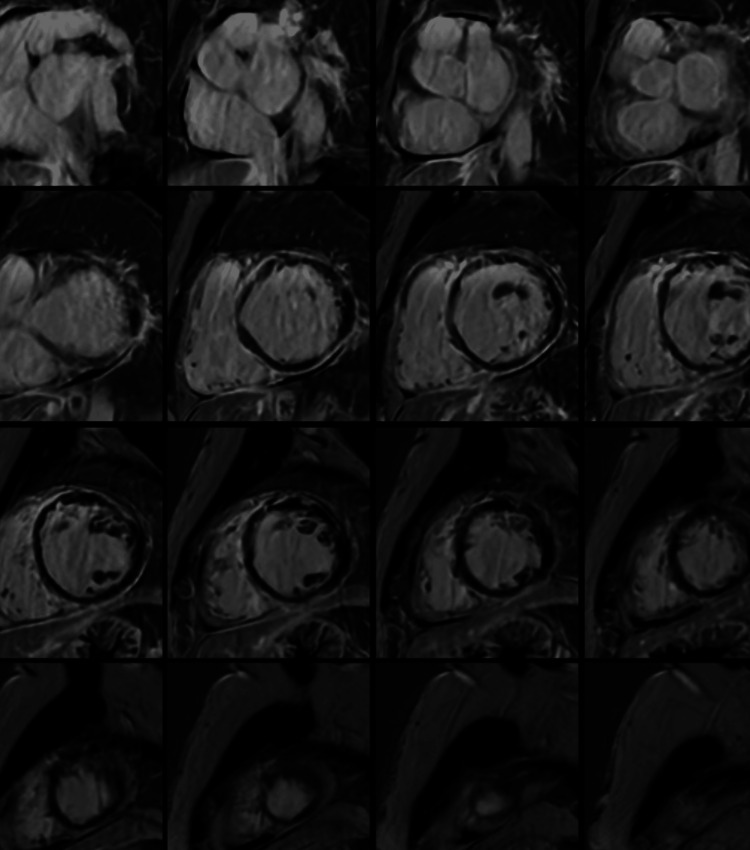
Gadolinium-enhanced cardiac MRI showing diffuse subepicardial and pericardial enhancement in addition to a severely dilated left ventricle with increased trabeculation and a mildly dilated right ventricle
